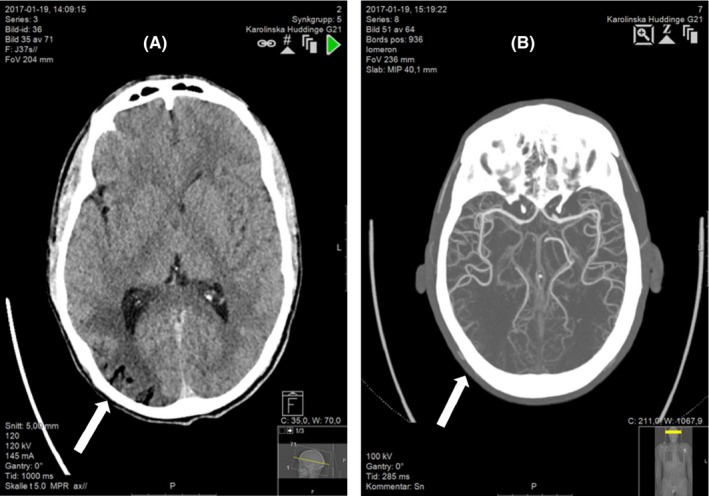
A, The scan showed gyriform air bubbles within the cortical arteries most pronounced in the fronto‐parieto‐occipital region in the right hemisphere, but also in the frontoparietal areas in the left hemisphere. The pattern was almost that of cortical watershed areas bilaterally, but most dominant on the right posterior side. B, The cervical and brain computed tomography angiography (CT‐AI) 70 min after the insult showed disappearance of air bubbles and no vessel occlusion. Noteworthy, but not stated in the initial report, there was a general thinning of arteries in the distal parietal areas, probably caused by an early reaction of the endothelial cells with swelling of the intima and narrowing of the arteries most severely affected by large bubbles of trapped air, as previously seen on the CT scan one hour earlier
